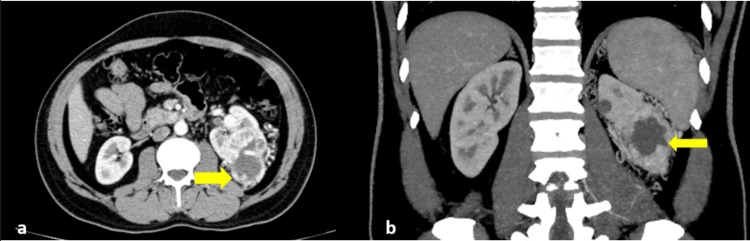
Contrast CT abdomen - axial (a) and coronal (b) images demonstrate enhancing centrally necrotic left renal mass (yellow arrows) CT: computed tomography
